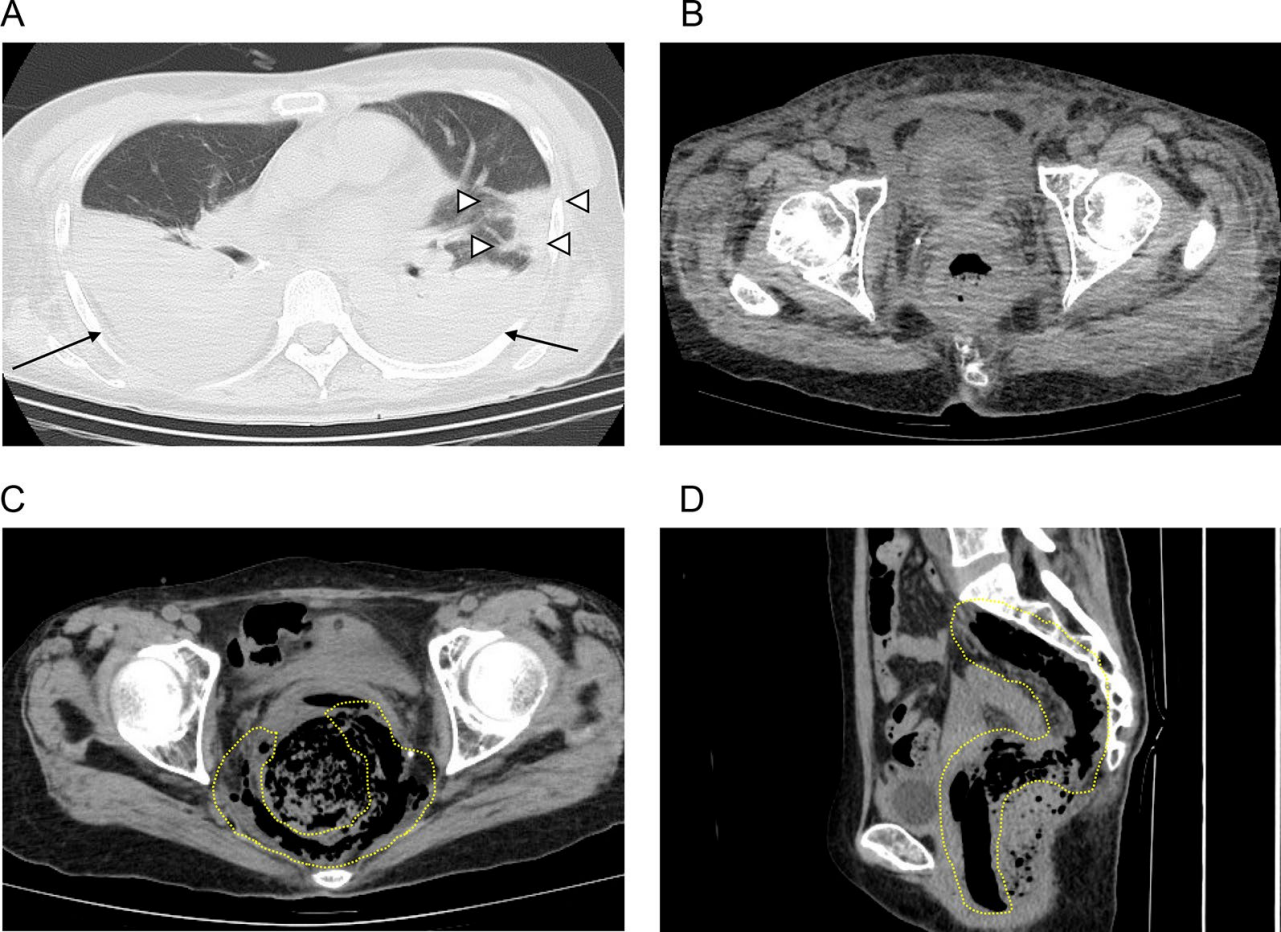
A, B CT imaging findings during COVID19 pneumonia. A Bilateral pleural effusion and a consolidation surrounded by ground-glass opacity in the left lung predominance were observed. Arrows indicate the pleural effusion. White arrowheads indicate the consolidation. B Rectum was slightly edematous. C, D CT imaging findings before emergency surgery. C (axial section) and D (sagittal section): free air was observed in the pelvic cavity. A yellow dotted line indicates the free air
